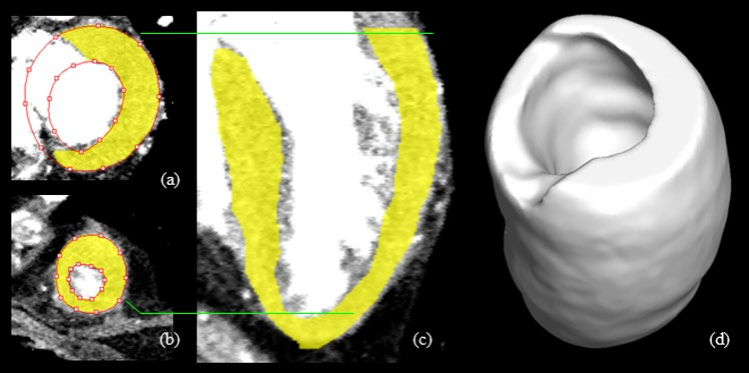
Overlay of the left ventricular segmentation (yellow) on CT: ((a), (b)) LV surface contour overlaid on axial sections with differences indicated (green lines indicate the level on the coronal section); (c) segmented LV mask overlaid on a coronal section; (d) 3D visualization of the LV.
